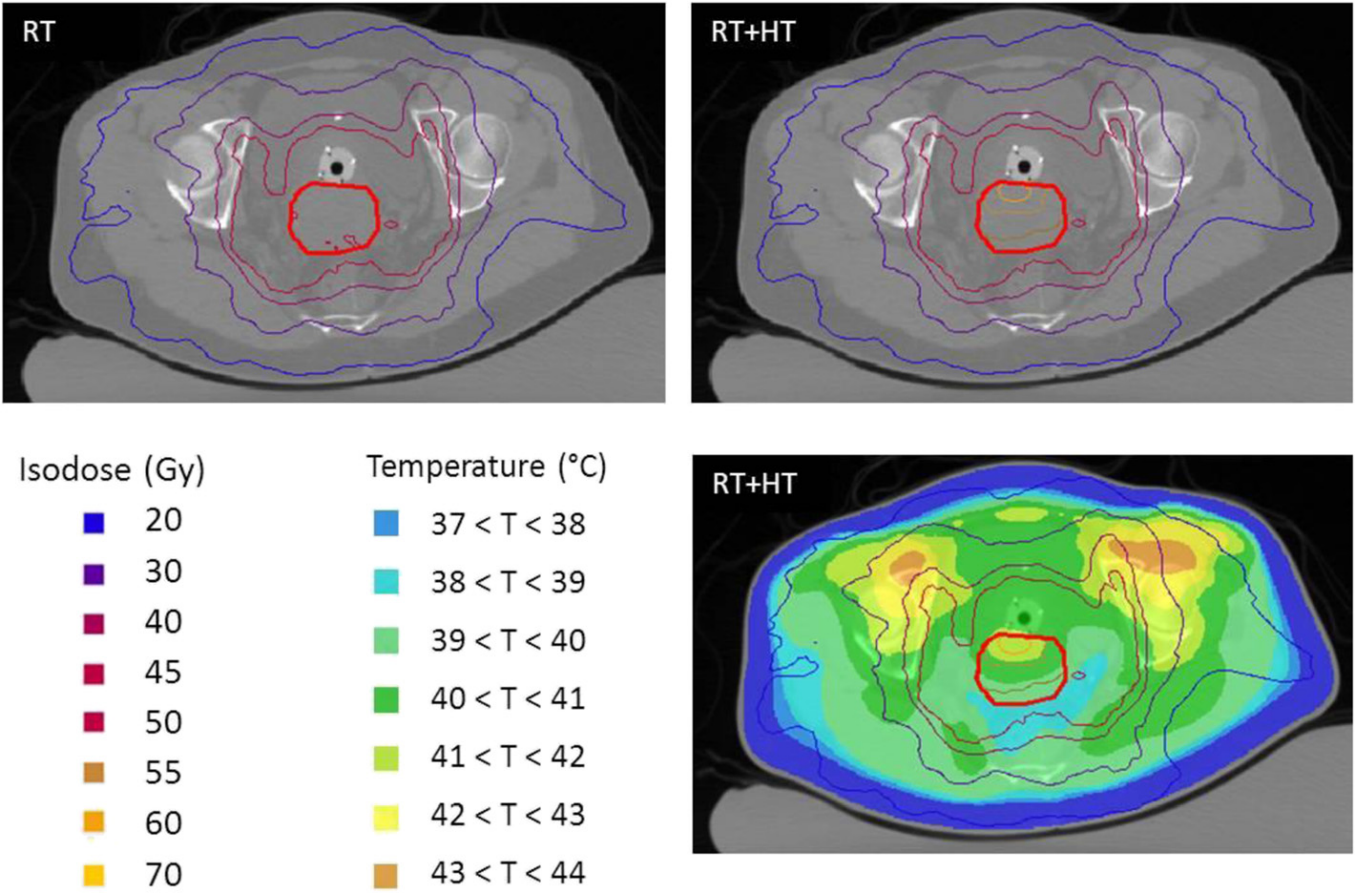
Radiotherapy and hyperthermia dose distributions. Radiotherapy isodose curves (top left), equivalent radiotherapy isodose curves for radiotherapy + hyperthermia (top right), idem with the hyperthermia temperature distribution overlaid as a color wash (bottom right) for patient #1 with cervical cancer. Radiotherapy isodose curves overlaid on the CT scan of the patient made in hyperthermia position lying on a hyperthermia water bolus. Gross tumour volume (GTV) is indicated by the bold red contour. The contribution of hyperthermia to equivalent radiotherapy isodose is visible within the GTV and increases with increasing temperature in ventral direction. Vaginal pelotte for temperature measurements during hyperthermia is visible adjacent to GTV
